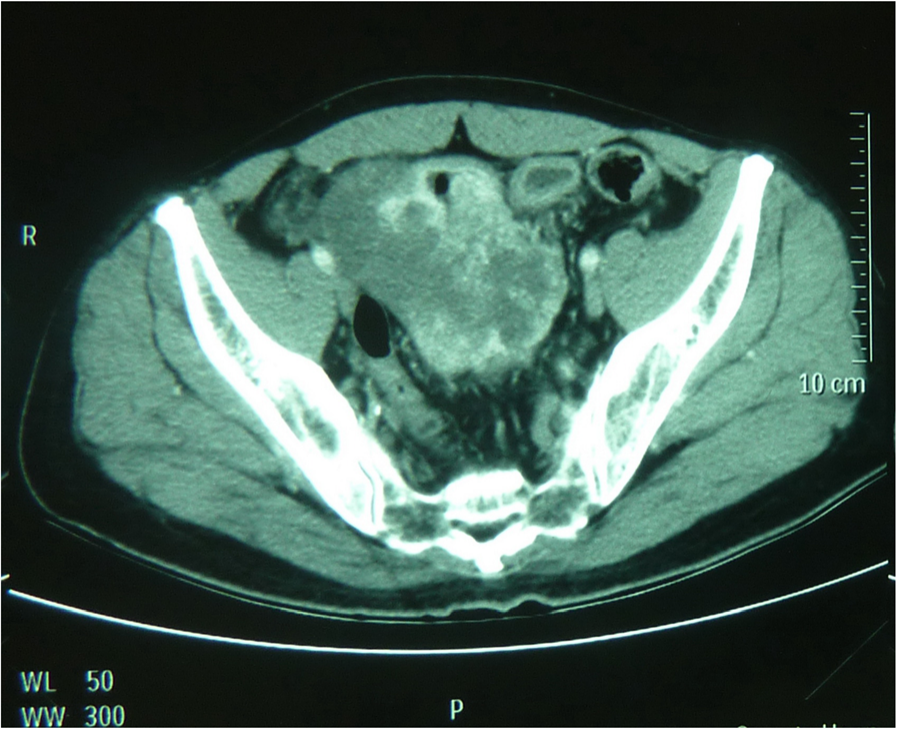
Imaging features of EIMS. Computed tomography (CT) scan revealed an enormous heterogeneous mass in the pelvic cavity
